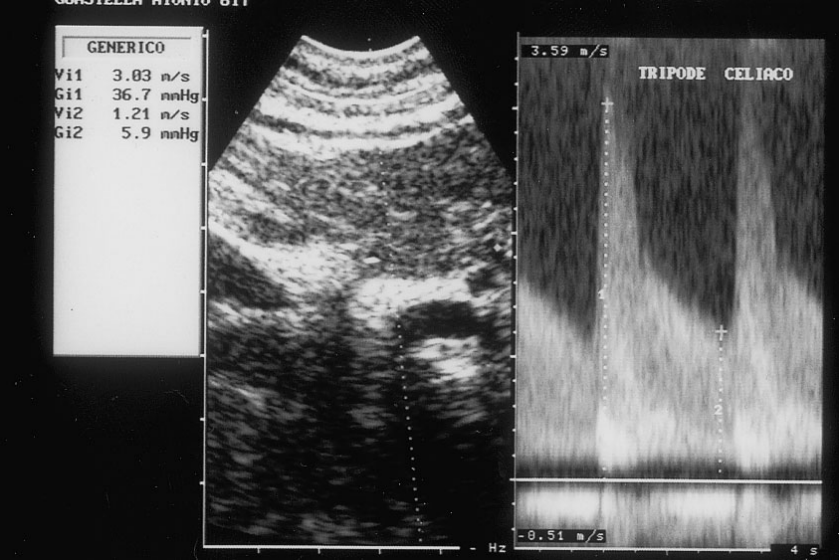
Continuous Doppler of Celiac Artery Stenosis.
